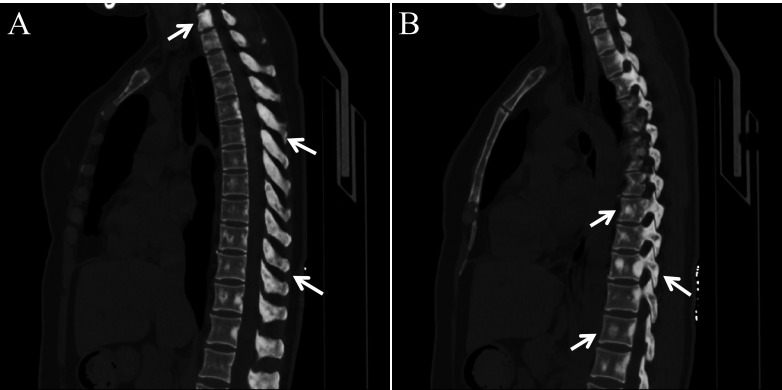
Sagittal chest computed tomography images (A, B) demonstrate multiple slightly hyperdense lesions in the patient’s spine (arrows).
